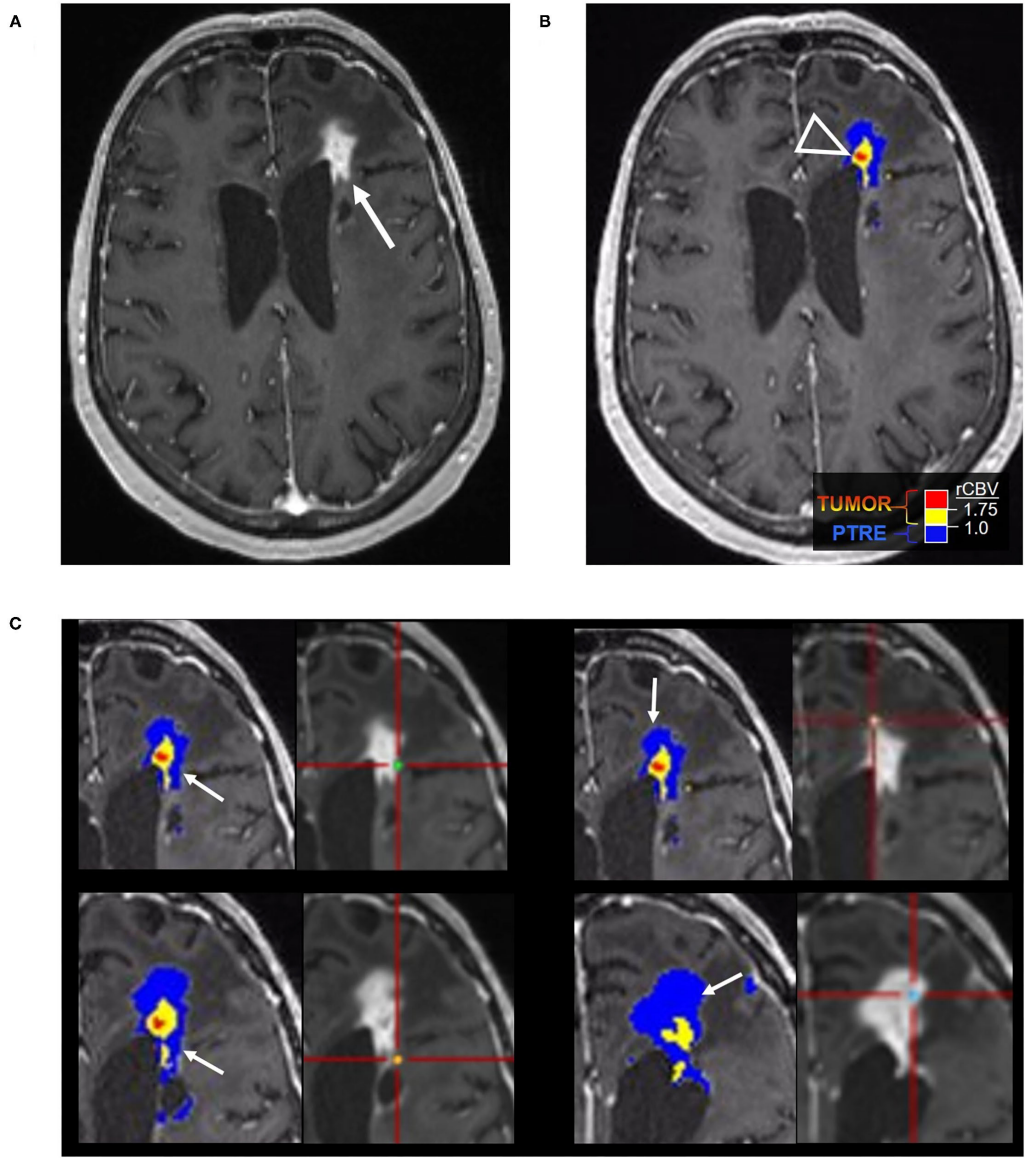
Posttreatment changes on DSC-MRI: A 68-year-old woman with left frontal GBM with two prior surgical resections and adjuvant radiation, temozolomide, and lomustine therapy undergoing routine surveillance imaging. (A) CE-MRI 2 years after initial diagnosis demonstrated increased nodular enhancement along the resection cavity concerning recurrent tumor. (B) The DSC FTB map derived from relative cerebral blood volume (rCBV) thresholds demonstrate a small area of high FTB centrally (arrowhead) suggesting tumor recurrence, but the majority of the surrounding tissue had low rCBV consistent with posttreatment effect. (C) Stereotactic biopsies were performed and cross-registered with areas of enhancement, but only corresponded with low rCBV regions. Pathology showed scant atypical cells in a background of extensive therapy-related changes, compatible with the rCBV map. No viable GBM was identified. Unfortunately, the central portion of the tumor was not sampled and cross-registration with the DSC images at the time of biopsy may have been helpful in fully characterizing the lesion pathologically.
